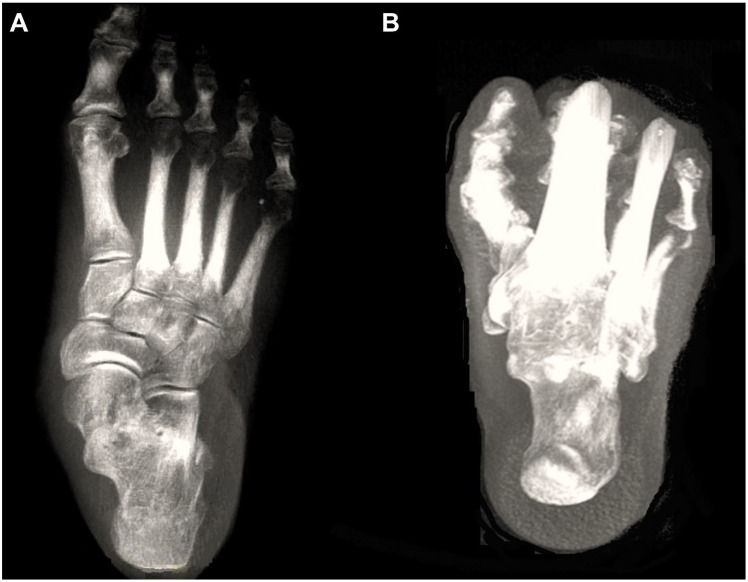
Digitally reconstructed radiographs (DRRs) demonstrating foot anteroposterior (A) and hindfoot alignment (B) views.
